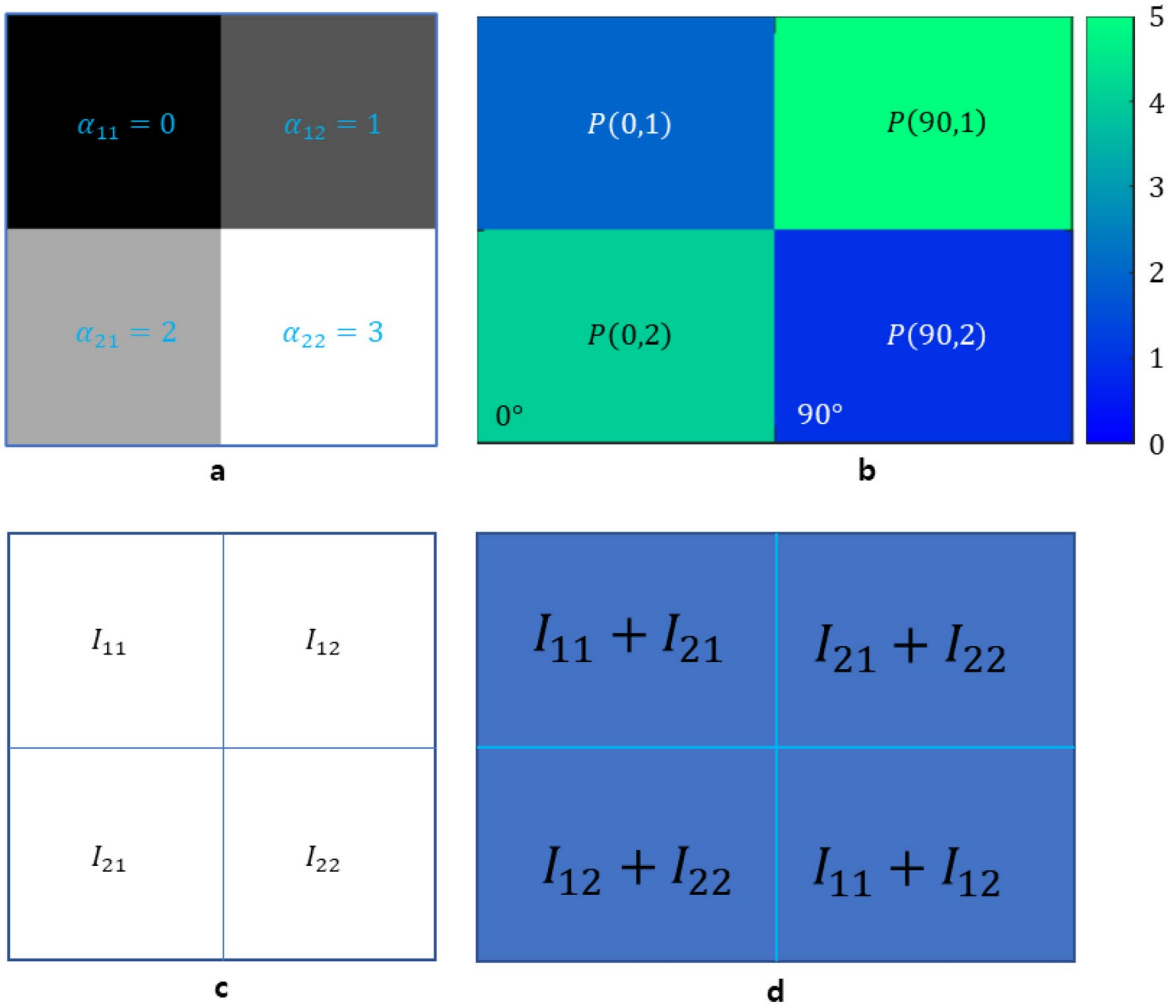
Example used in the QUBO model. (a) $$2\times 2$$ image sample with given mass attenuation coefficients. (b) A sinogram coming from the image sample in (a). (c) An undetermined CT image with qubit variables. (d) An undetermined sinogram with two projection angles of 0 and 90° by the Radon transform of the undetermined CT image in (c).
